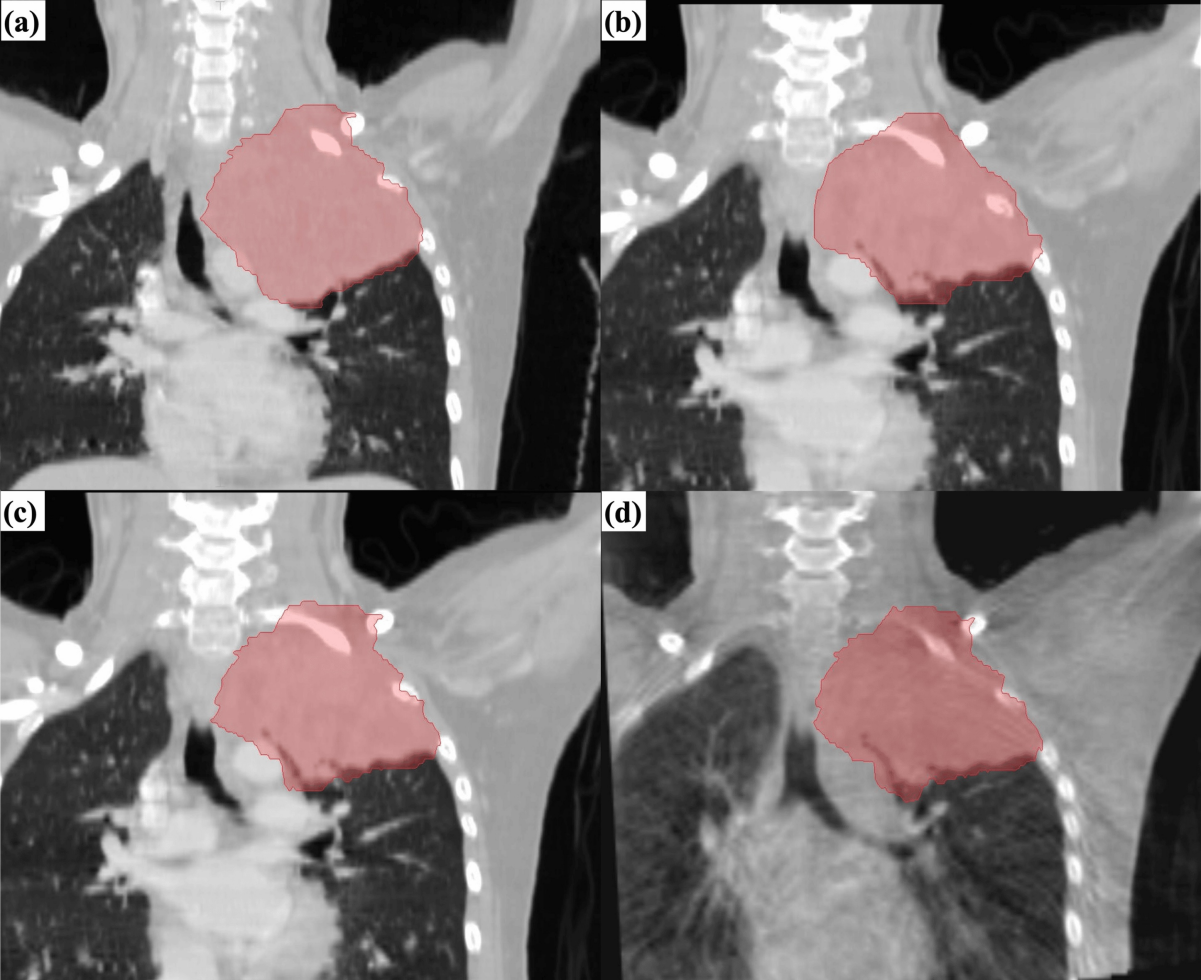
An example of the gross tumor volume structures contoured by Adapt Anatomy for a patient with lung cancer (a) Planning computed tomography contoured by radiation oncologists; (b) Replanning CT contoured by radiation oncologists; (c) Replanning CT contoured automatically by Adapt Anatomy; (d) Cone-beam computed tomography contoured automatically by Adapt Anatomy
